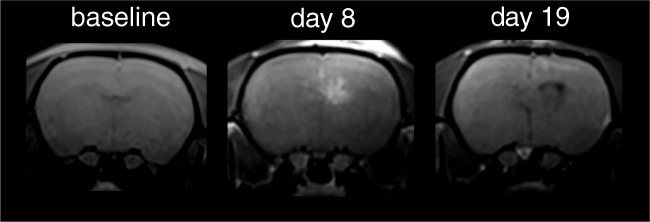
MR images before GS9L cell inoculation (baseline) 1 week after inoculation (day 8) and at the end of the study (day 19). On day 8, the tumor showed a strong signal enhancement 1 h after the administration of gadopentetate dimeglumine. An increased lesion size and a midline shift were found on the MRI acquired 1 week after the last administration (day 19)
